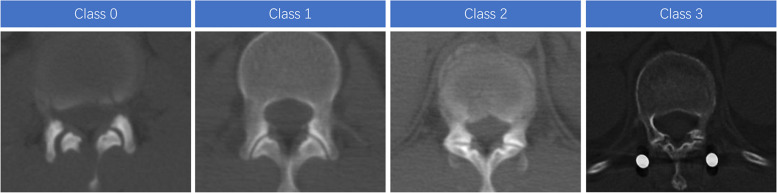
Representative examples of CT scans demonstrating the different classes of facet joints degeneration
